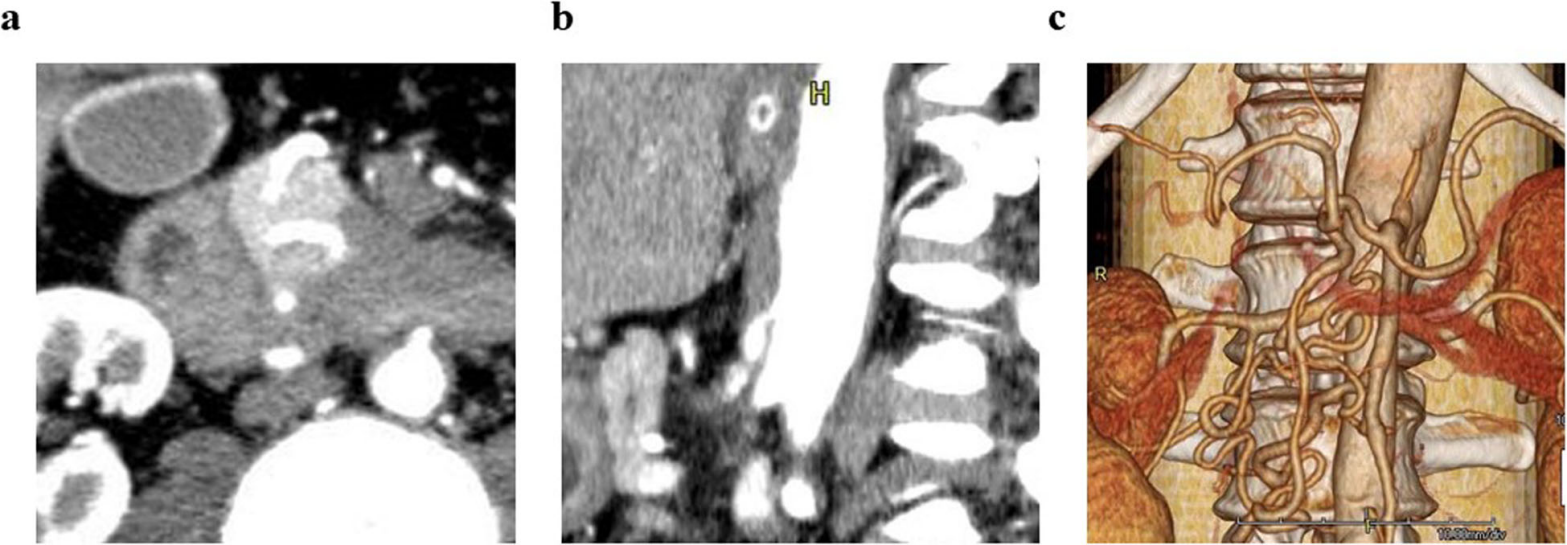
Preoperative enhanced CT (case 1). a Low-density area surrounding normal tissue of the pancreas and duodenum. b CACS due to MALS. Hooked appearance and minimal post-stenotic dilatation. c 3D reconstruction image of blood vessels. Inferior pancreaticoduodenal artery was dilated and tortuous
